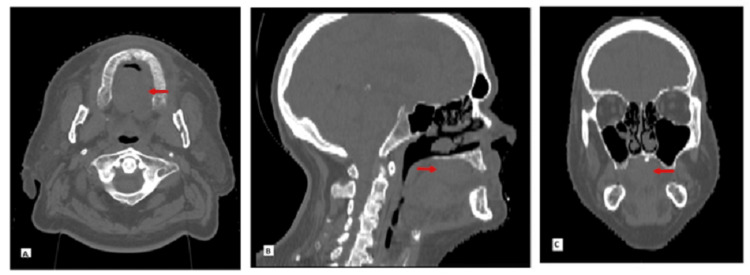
Non-contrast CT of sinuses and face: (A) Transverse section, (B) Sagittal section, and (C) Coronal section demonstrating hard palate mass (red arrow) with bony destruction
